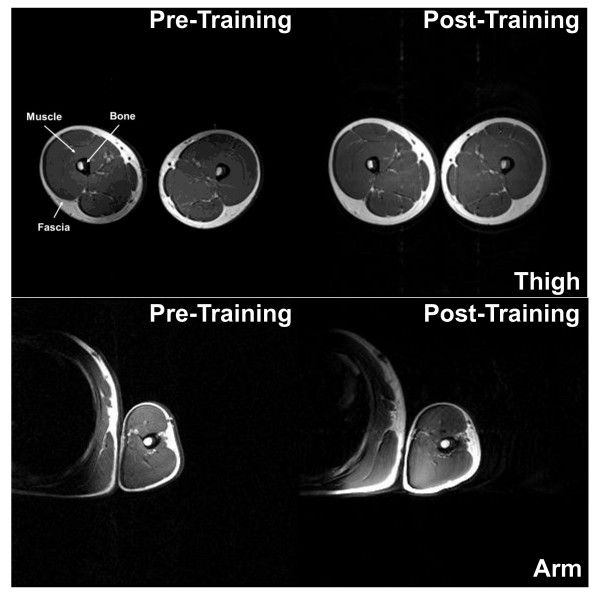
Magnetic resonance images of the right thigh and upper arm for a single subject pre- and post-training. Thigh and arm scan were obtained using axial T1-weighted spin-echo images with repetition time of 750 ms, echo time of 20 ms, 230 × 290 matrix resolution and number of excitations of two. Thigh images were obtained perpendicular to the femur starting at the proximal femoral epiphysis (tangential to its proximal end) and proceeding distally toward the knee joint. The slice thickness was 8 mm with no gap (forty slices) with a 45 × 45 cm field of view (FOV). Upper arm images were obtained perpendicular to the humerus starting at the proximal humeral epiphysis (tangential to its proximal end) proceeding distally toward the elbow joint. The slice thickness was 6 mm with a 1.2 mm interslice gap (forty slices) with a FOV of 40 × 32 or 40 × 40 cm depending on the arm's size.
